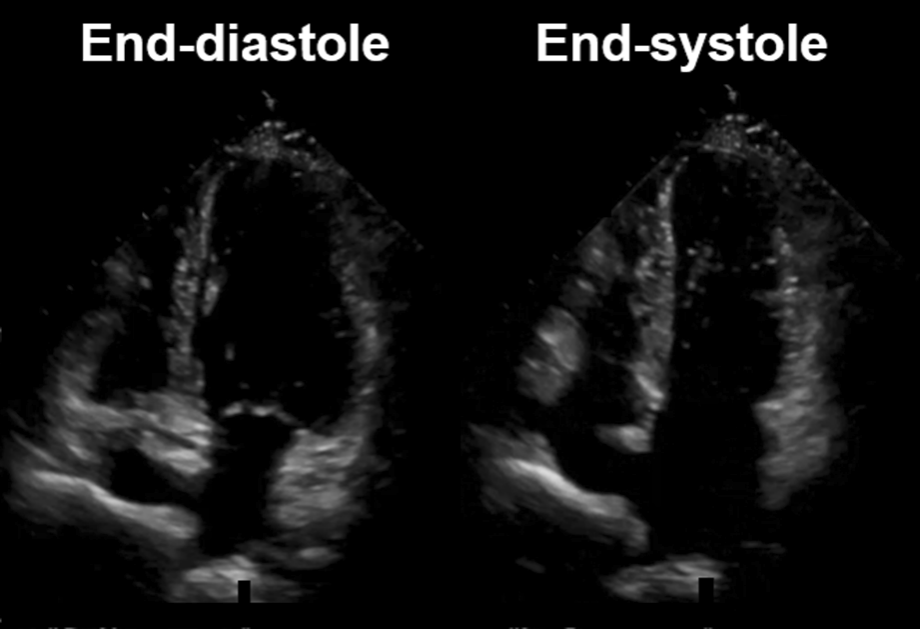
Apical four-chamber view of transthoracic echocardiographic images in diastole and systole 2 weeks after discharge. Echocardiographic imaging demonstrating normal LV wall motion and trivial apical hypertrophy with estimated LV ejection fraction of 63%
